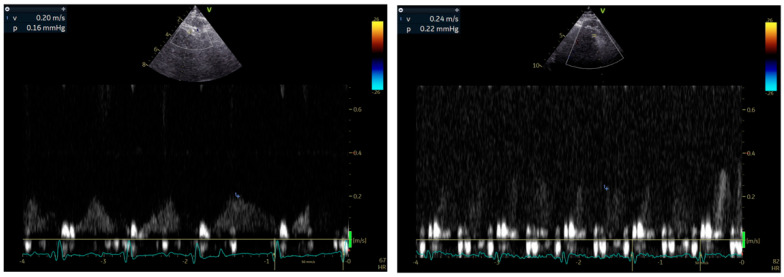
Representative example of CFR calculation using echocardiography.
